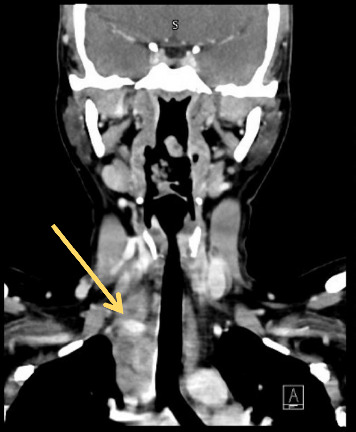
Neck CT with contrast: conglomerate of enlarged necrotic lymph nodes seen in the right paratracheal region/superior mediastinum (yellow arrow), with no evidence of compression on the right IJV.
